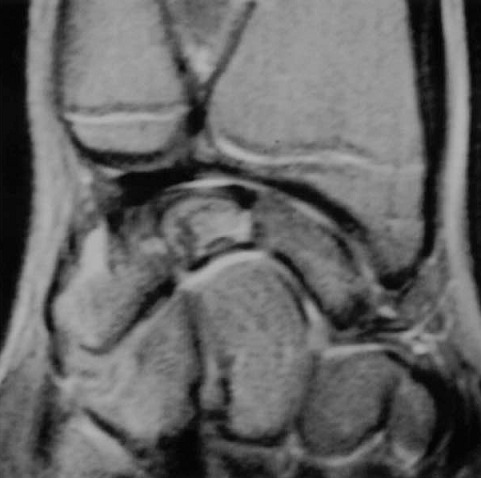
Magnetic resonance imaging (MRI) shows beginning of revascularization of the lunate
